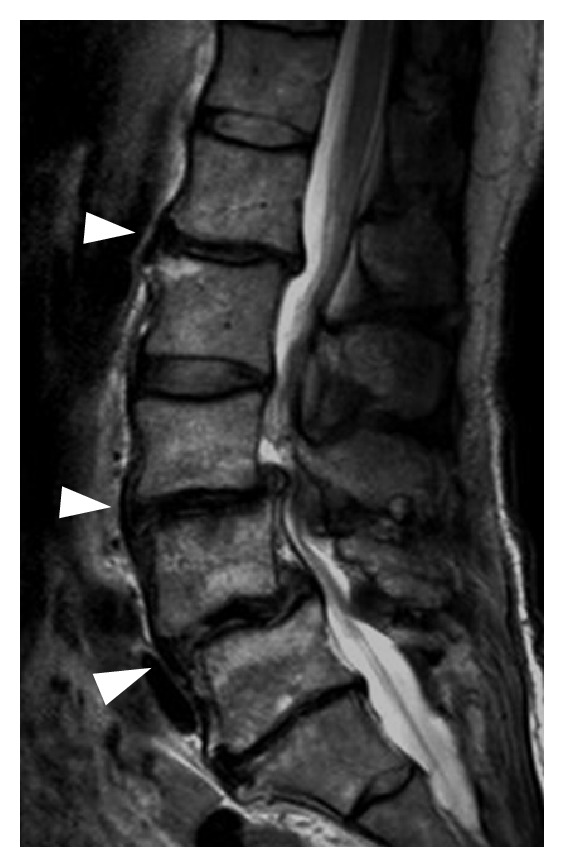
Sagittal T2-weighted magnetic resonance image before the spinal fusion operation showing severe disc degeneration (arrowhead) at the L1/2, L3/4, and L4/5 levels.
